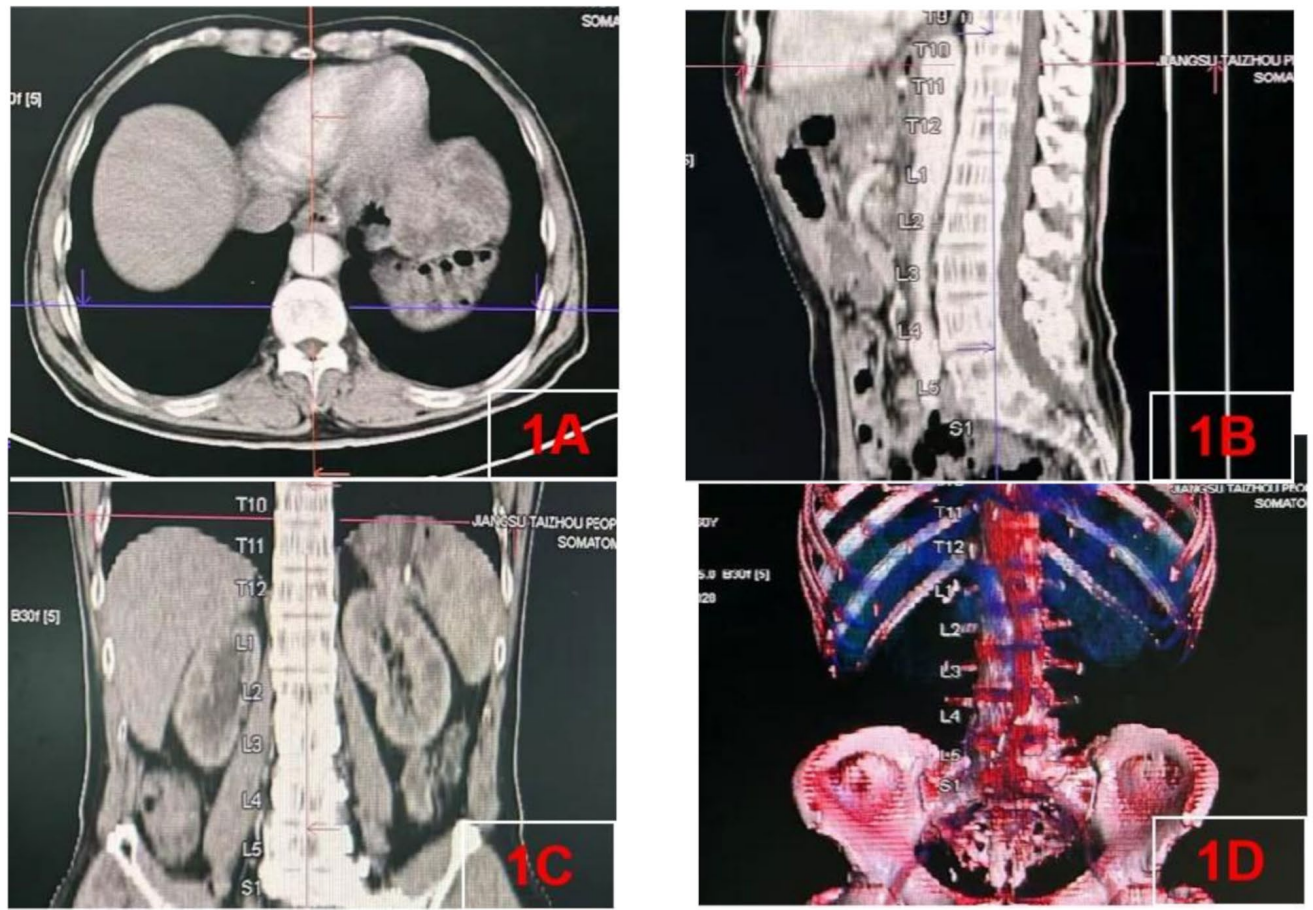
CT images: A, cross-sectional (axial) images of the thoracolumbar and lumbar spine; B, sagittal plane visualization of the thoracolumbar and lumbar region; C, coronal plane anatomical sections through the thoracolumbar and lumbar vertebrae; D, three-dimensional reconstructed volumetric rendering of the thoracolumbar and lumbar spine
